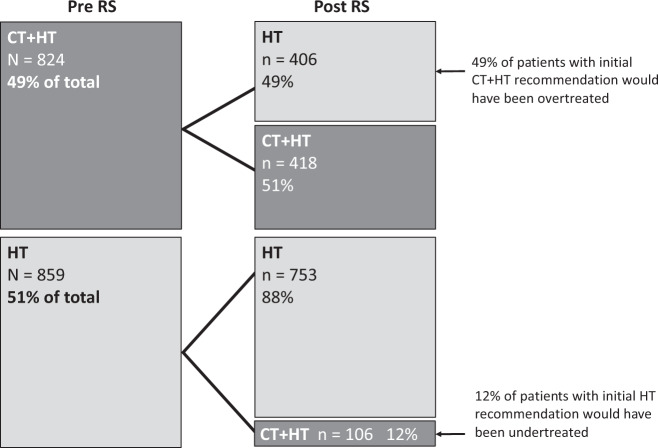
Changes in treatment recommendations before and after availability of the Recurrence Score result. Rates of hormone therapy alone (HT) or chemo-endocrine therapy (CT+HT) recommendations before testing (Pre RS) and changes in recommendations based on the test resutls (Post RS).
